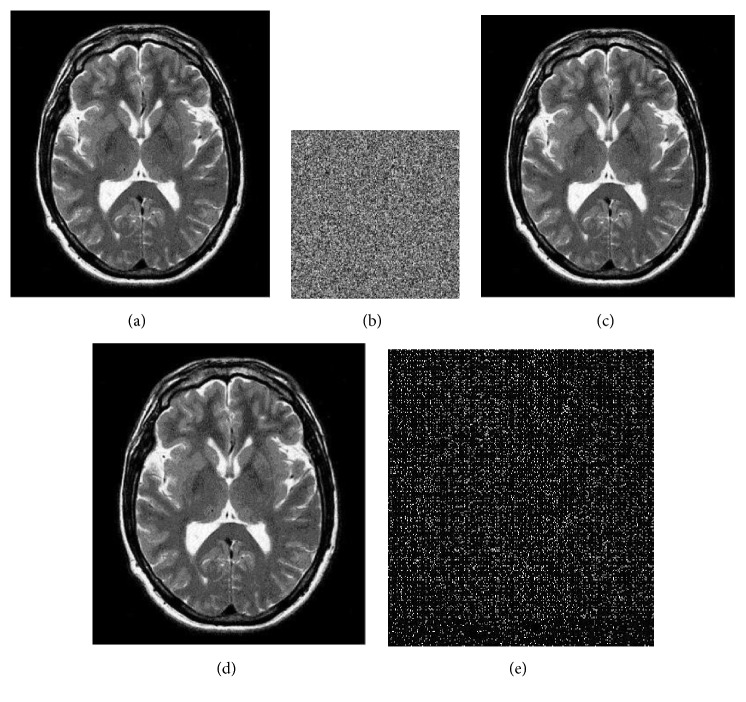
(a) Original MRI image; (b) chaotic watermark; (c) watermarked image. (d) Recovered image with exact initial conditions. (e) Recovered image with x(0) = 0.25000001, r = 3.58, and x(0) = 0.56, r = 3.57.
